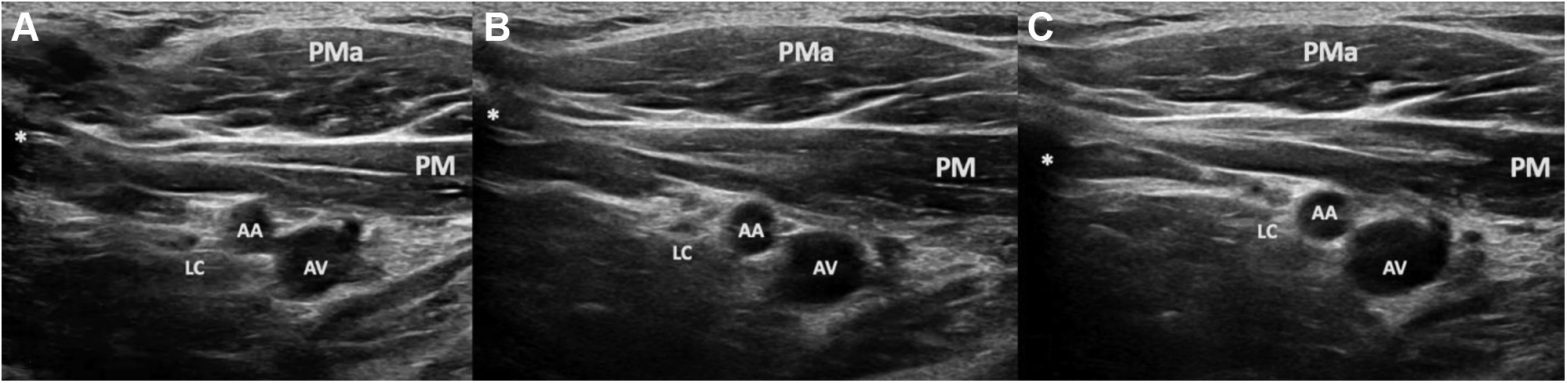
Dynamic ultrasound evaluation of the right pectoralis minor during active arm abduction in the plane of the body, in a normal patient without symptoms. (A) Resting adduction with arm at the side. (B) 90^o^ active abduction. (C) 120^o^ active abduction. Note the absence of posterior pectoralis minor muscle indentation. *AA*, axillary artery; *AV*, axillary vein; *LC*, lateral cord; *PM*, pectoralis minor; *PMa*, pectoralis major; ∗ indicates coracoid.
